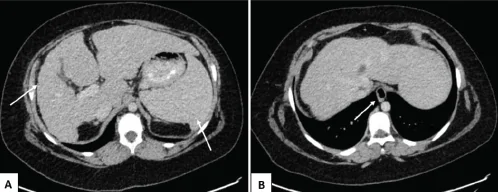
Computed tomography abdomen with intravenous and oral contrast demonstrating: (A) Irregular hepatic contour consistent with cirrhosis and splenomegaly (arrows), suggestive of portal hypertension. (B) Dilated gastroesophageal varices (arrow), consistent with portal hypertension.
